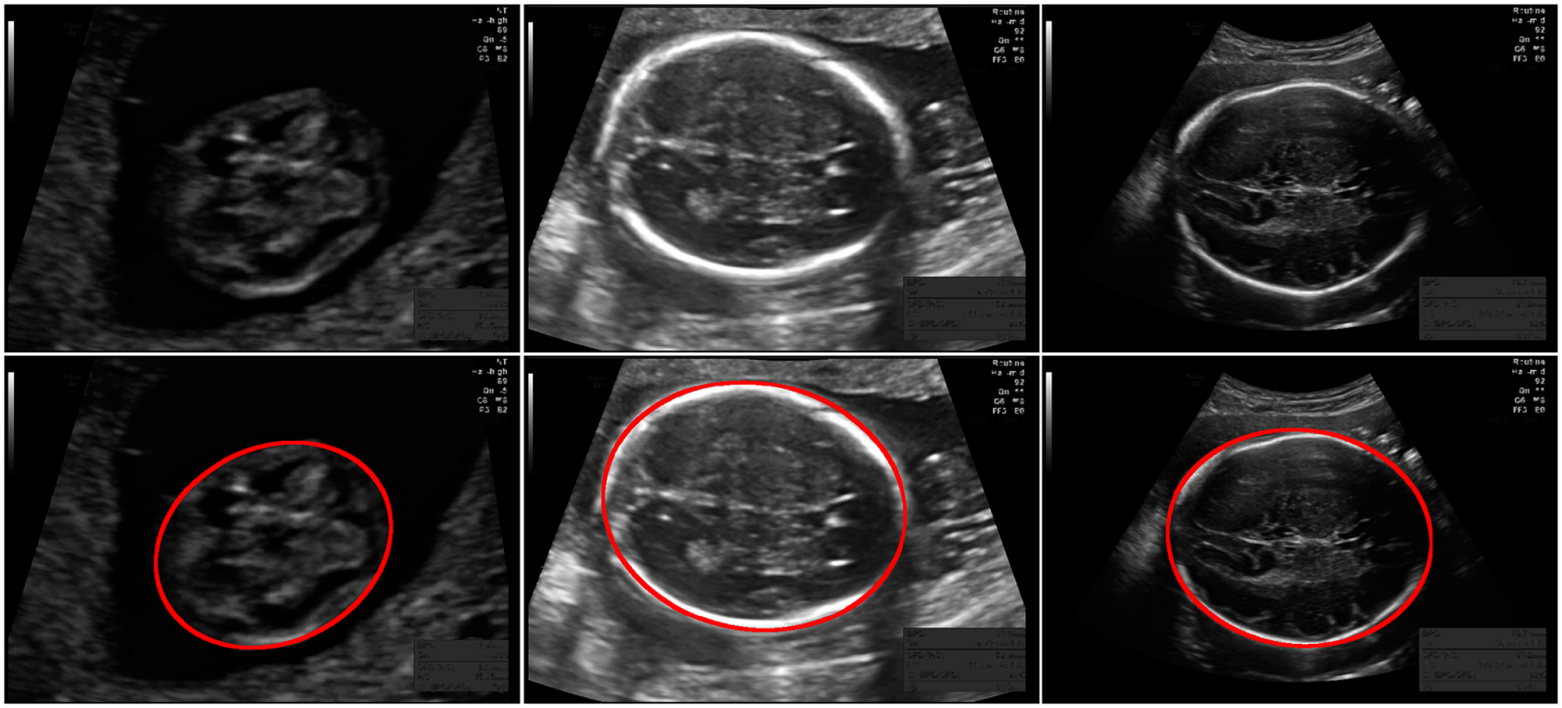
Example ultrasound images. From top to bottom: without annotation and with annotation in red. From left to right: first trimester with an HC of 65.1 mm (pixel size of 0.06 mm), second trimester with an HC of 167.9 mm (pixel size of 0.12 mm) and third trimester with an HC of 278.4 mm (pixel size of 0.24 mm). Note that the skull is not yet visible as a bright structure in the first trimester.
